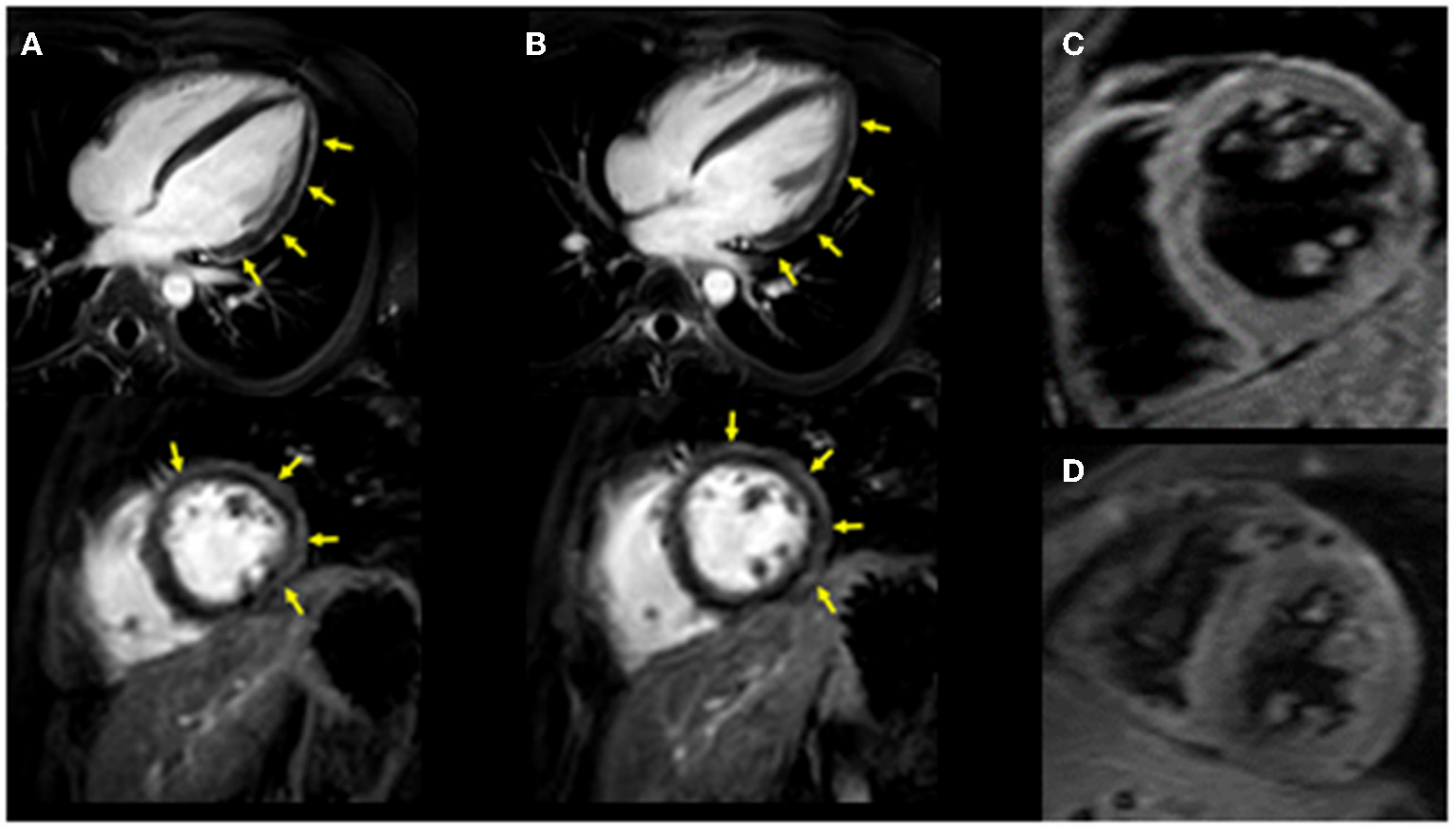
Cardiac MRI imaging. Diffuse late gadolinium enhancement at the epicardium was observed in both images. (A) Images obtained 3 years ago when he suffered from his previous myocarditis (top: long-axis view, bottom: short-axis view). (B) Images obtained during the current myocarditis episode associated with coronavirus disease-2019 (COVID-19) messenger RNA (mRNA) vaccination (top: long-axis view, bottom: short-axis view). T2-weighted MR images. (C) Image obtained 3 years ago when he suffered from his previous myocarditis episode. (D) Image obtained during the current myocarditis episode associated with COVID-19 mRNA vaccination.
